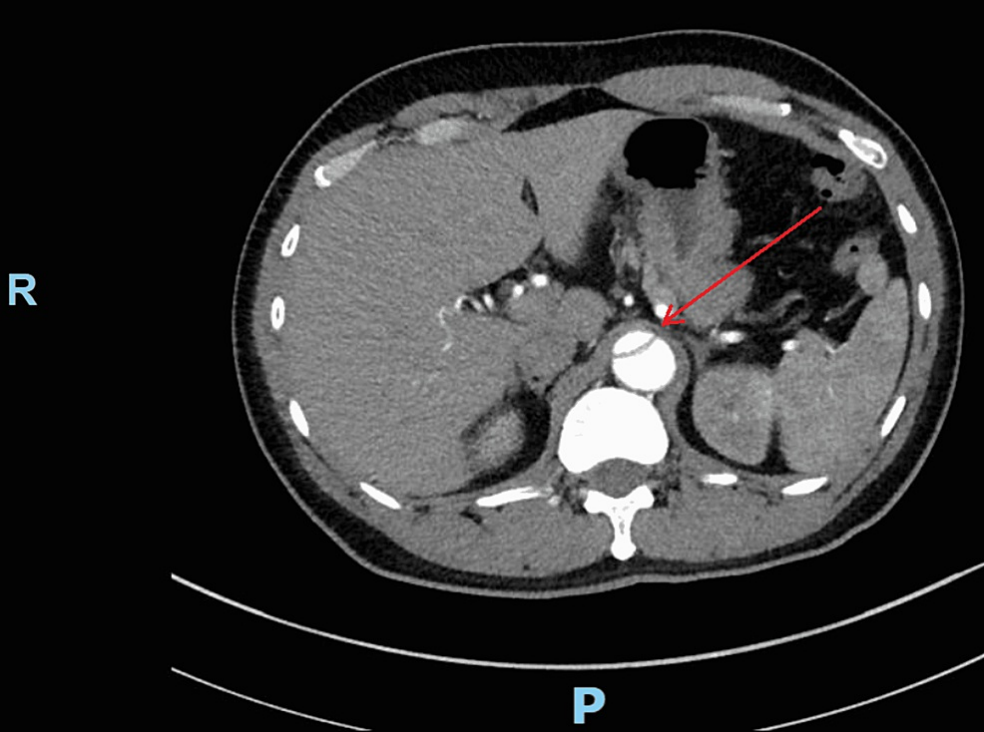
Axial CT angiography of the abdomen with contrast showing aortic dissection
